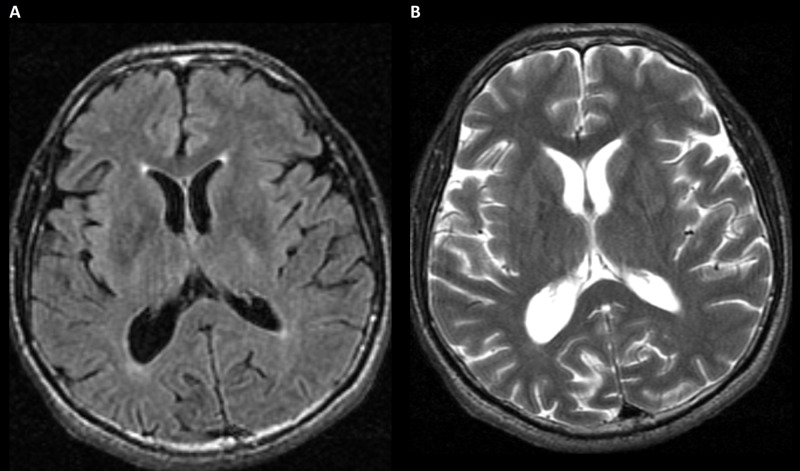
MRI upon admission. (A) FLAIR image. (B) Axial T2 image. There are no hyperintensity signals in the basal ganglia or hippocampal regions. FLAIR, fluid-attenuated inversion recovery
